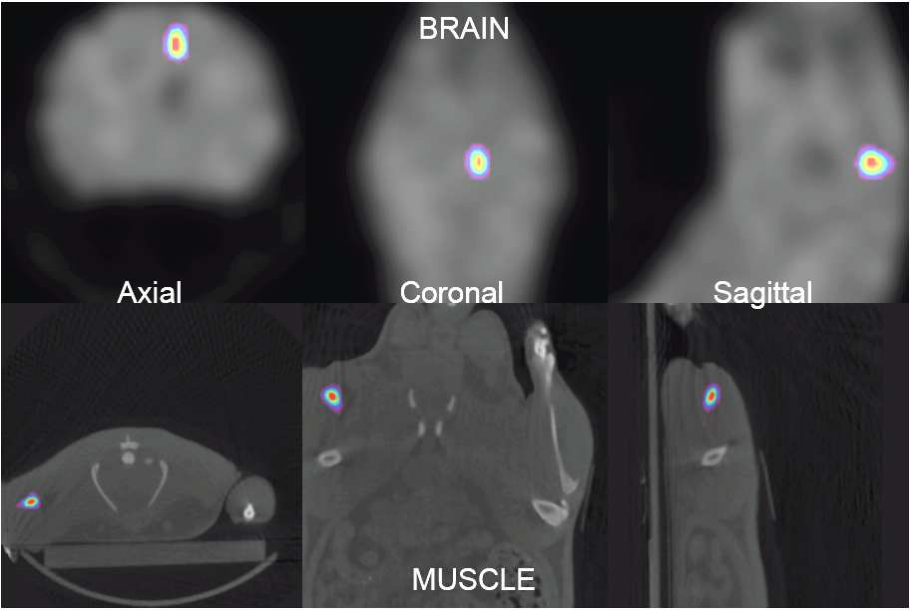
Axial view of the ^11^C activity distributions at the beginning of PET acquisition (in the brain at the top, and in the muscle at the bottom). PET images were fused with X ray CT scan for a better injected point localization.
